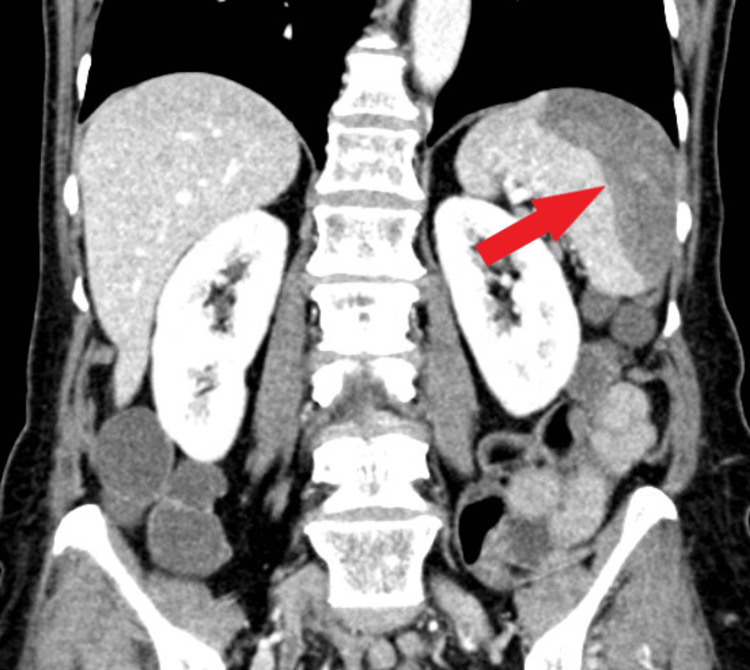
CT abdomen coronal section showing subcapsular splenic hematoma (red arrow).
